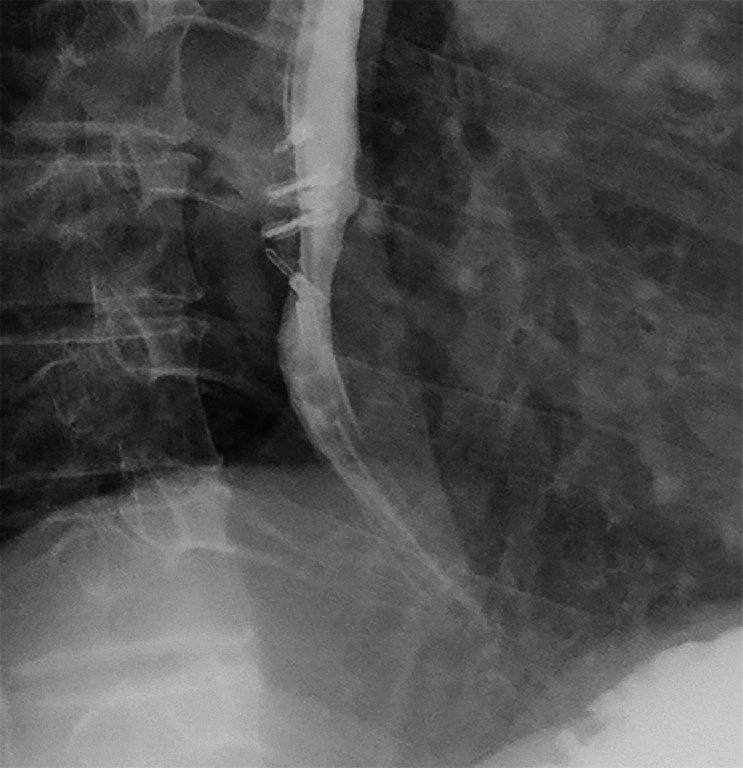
After 48 hours, upper gastrointestinal radiography showed resolution of the mid-esophageal diverticulum, and no contrast agent extravasation was observed.
